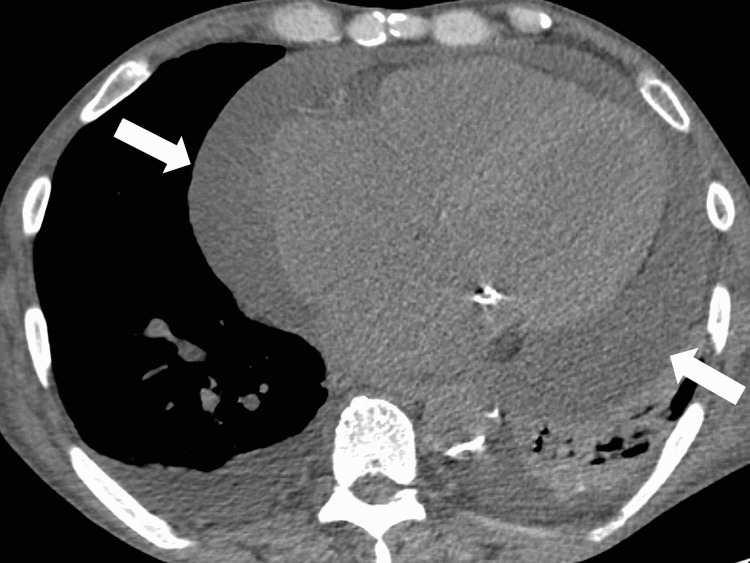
Cardiac tamponade on the subsequent computed tomography
